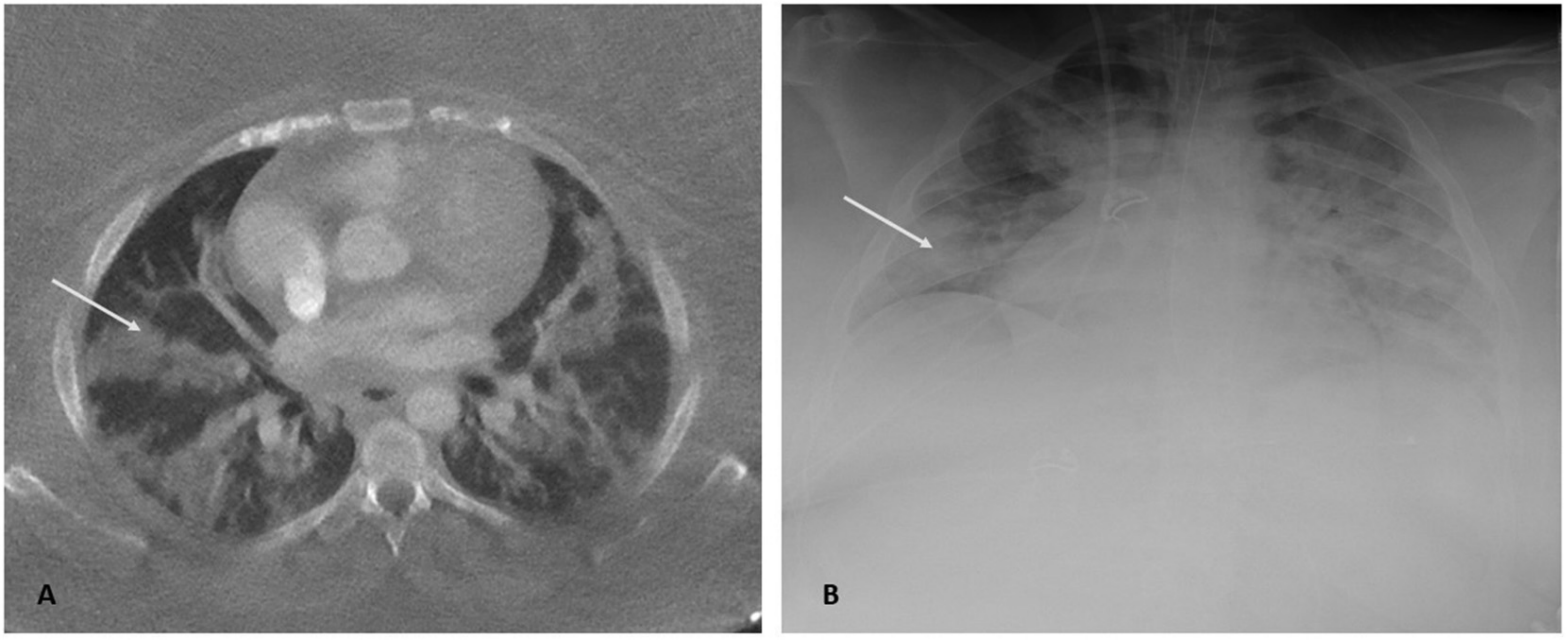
A. Low-dose computed tomography axial scan showing bilateral multifocal ground-glass opacities, with both peripheral and perihilar distribution, corresponding to COVID-19 pneumonia. B. Chest radiograph showing bilateral opacities and signs of congestion.
